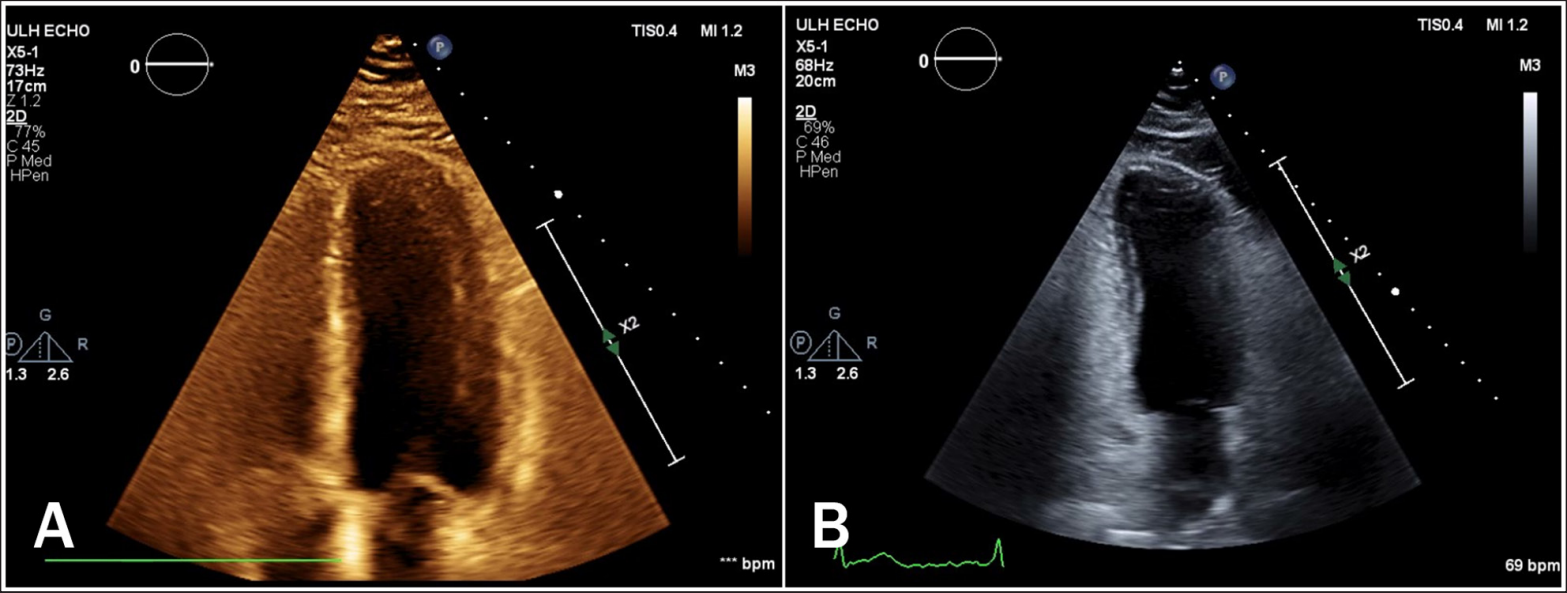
Follow-up transthoracic echocardiography with recovered left ventricular function in (A) apical 4-chamber and (B) apical 2-chamber views.
